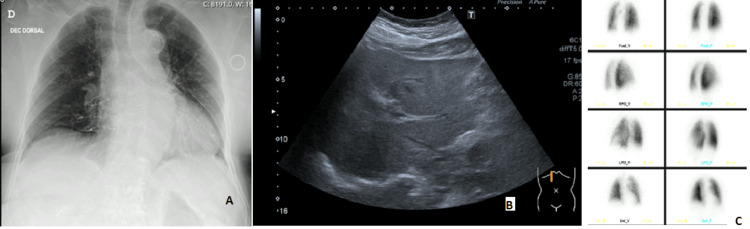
Patient's chest radiography (A), upper abdominal ultrasound (B), and CT pulmonary angiogram (C) The normality of the findings of these CDEs allowed the exclusion of post-pneumonectomy status, chronic liver disease, and pulmonary vascular diseases as causes of POS. CDE: complementary diagnostic exam; POS: platypnea-orthodeoxia syndrome
